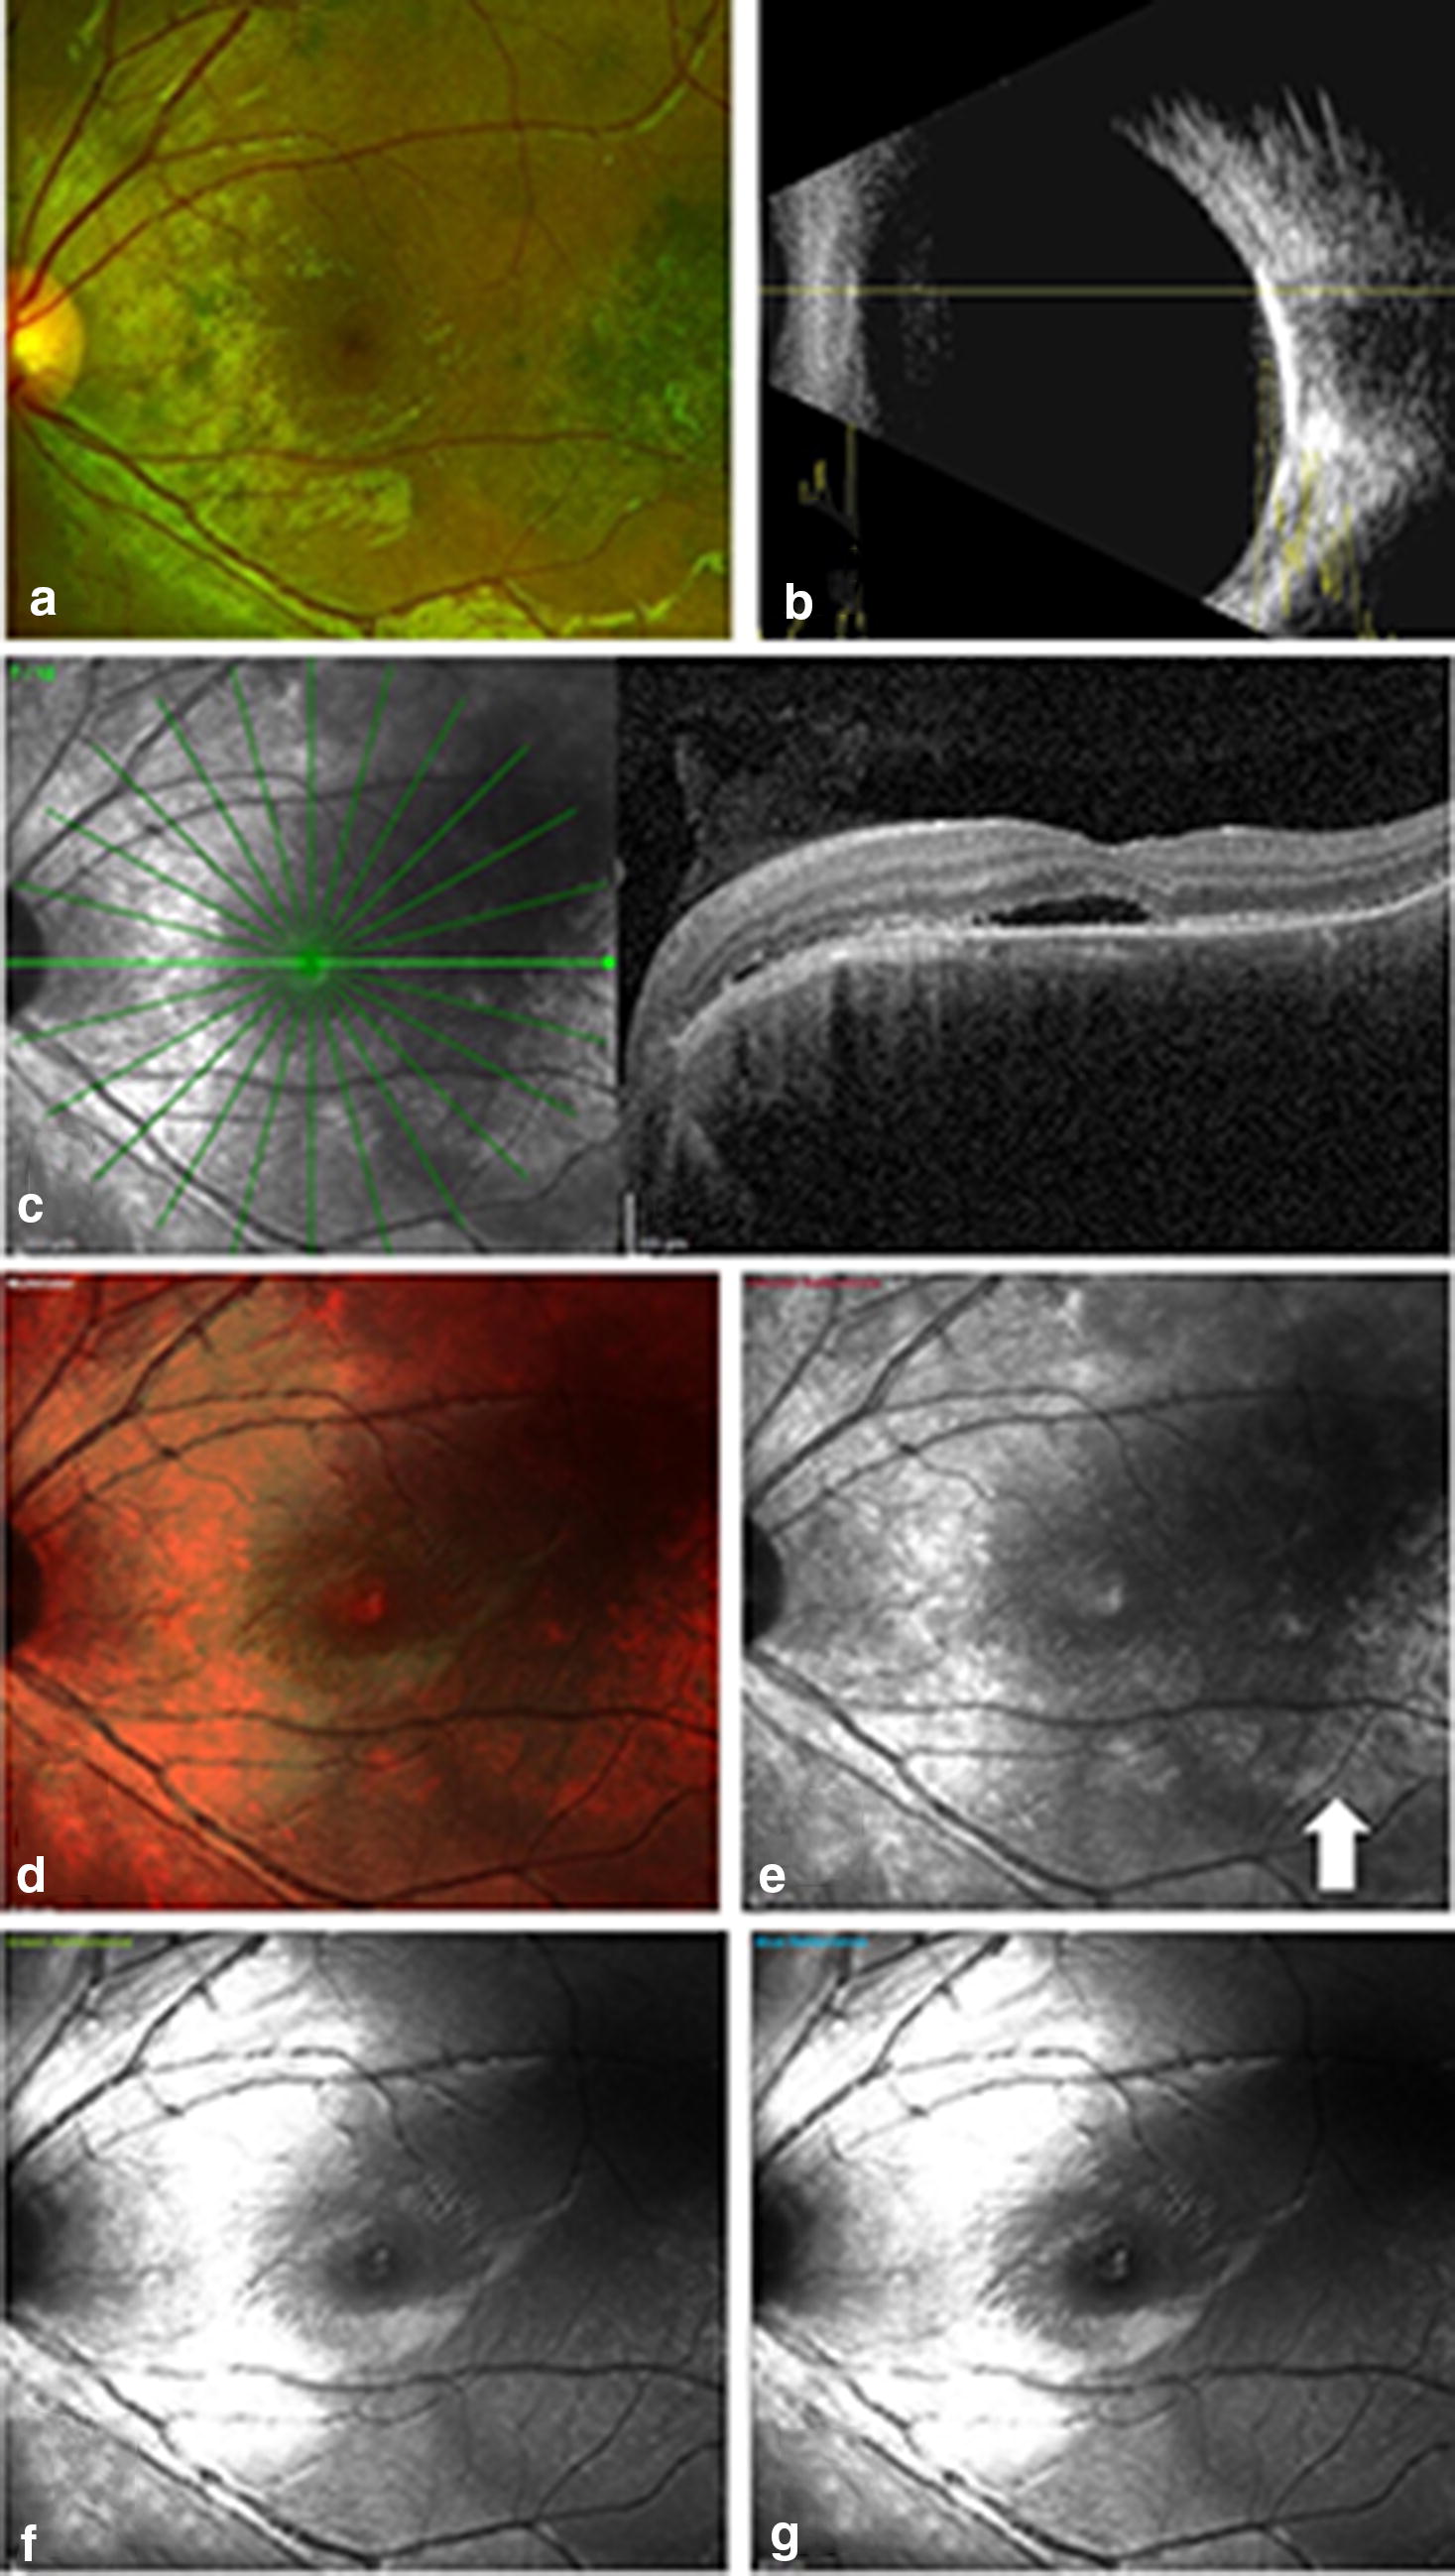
Case 3—Multimodal imaging in a patient with predominantly decalcified choroidal osteoma in the LE. a CF showing yellowish choroidal osteoma lesion at the peripapillary region with retinal pigment epithelial alterations noted temporal to the macula. b Ocular ultrasound showed hyperechoic area with shadowing confirming CO. c Horizontal line OCT scan passing through the fovea shows subtle elevation by the tumour temporal to the ONH and presence of sub foveal SRF. d MC image shows minimal orangish discoloration in the peripapillary area and temporal to the macula suggestive of overlying RPE atrophy. The decalcified tumour is not seen on MC image. e Infrared reflectance shows white areas corresponding to the orange areas in MC image suggestive of atrophied RPE. f, g Blue and green reflectance images do not show the osteoma lesion
